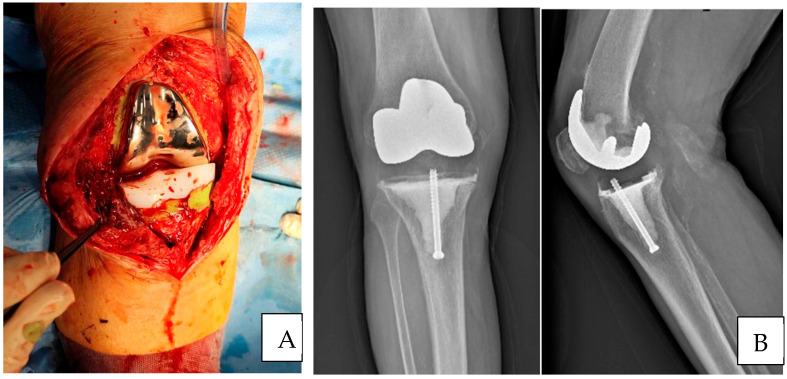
Final implantation and postoperative radiograph. (A) During cement fixation, the lower limb should be maintained in extension with constant traction to prevent the development of varus/valgus deformities or soft tissue shortening caused by contact between the femoral shield and the polyethylene insert. (B) The anteroposterior and lateral postoperative radiographs show a stable implant with adequate filling of bone defects through cementation.
